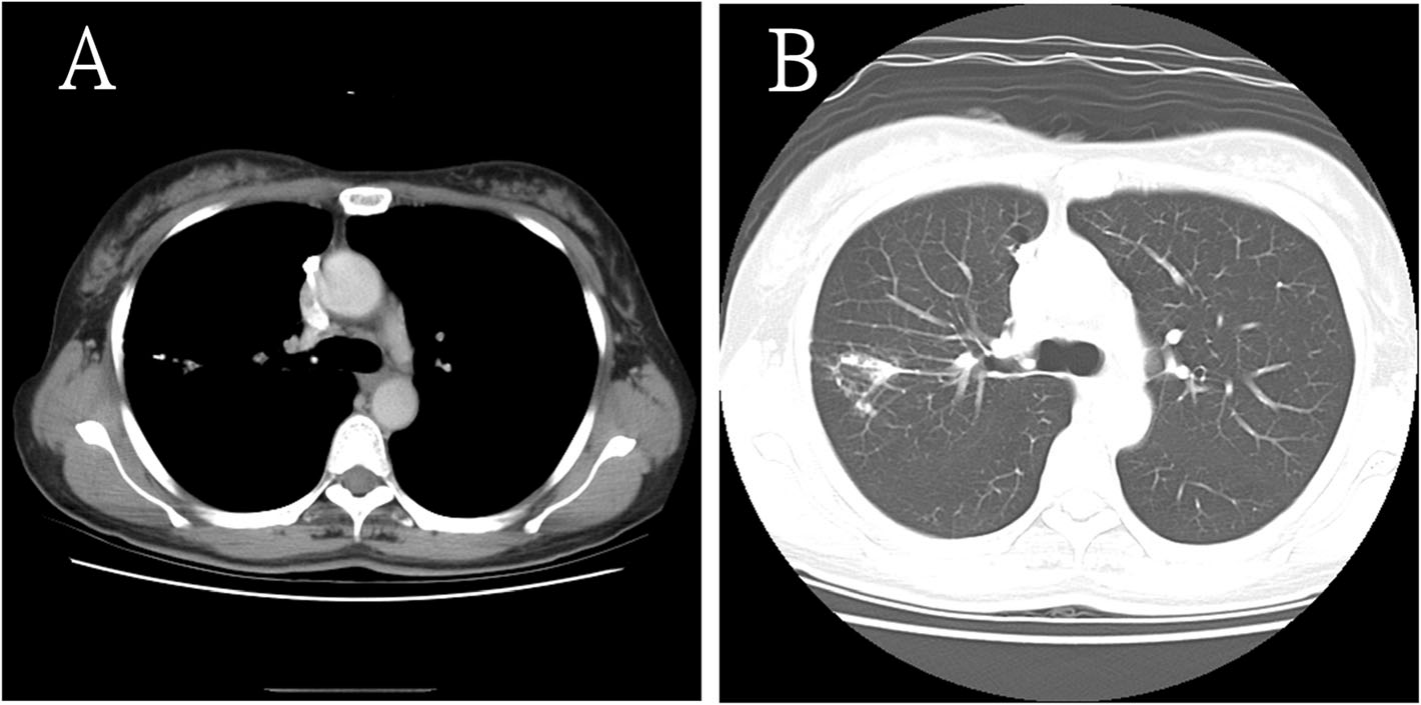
Follow-up chest computed tomography performed 2 years later. Two year later, chest computed tomography shows that the calcified nodule has “melted” over time and remains encased in the dilated bronchus (yellow arrowhead) (a). It is accompanied by aggregating parenchymal infiltration (blue arrowheads) (b)
